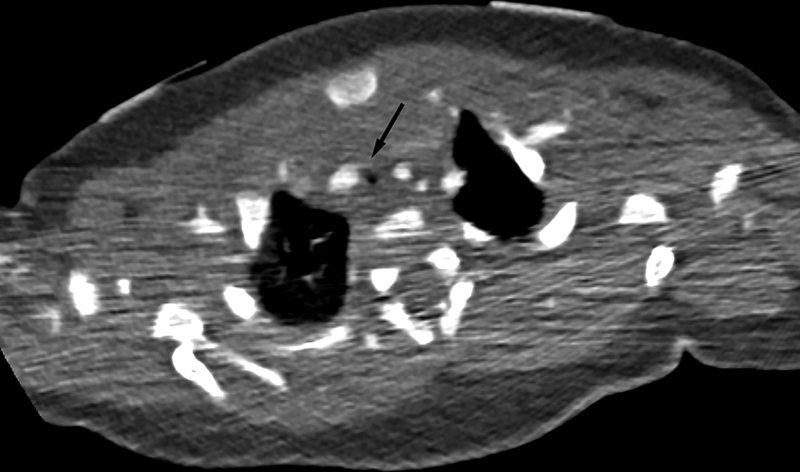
Axial chest computed tomography with intravenous contrast shows marked narrowing of the trachea (arrow) with the innominate artery to the right.
